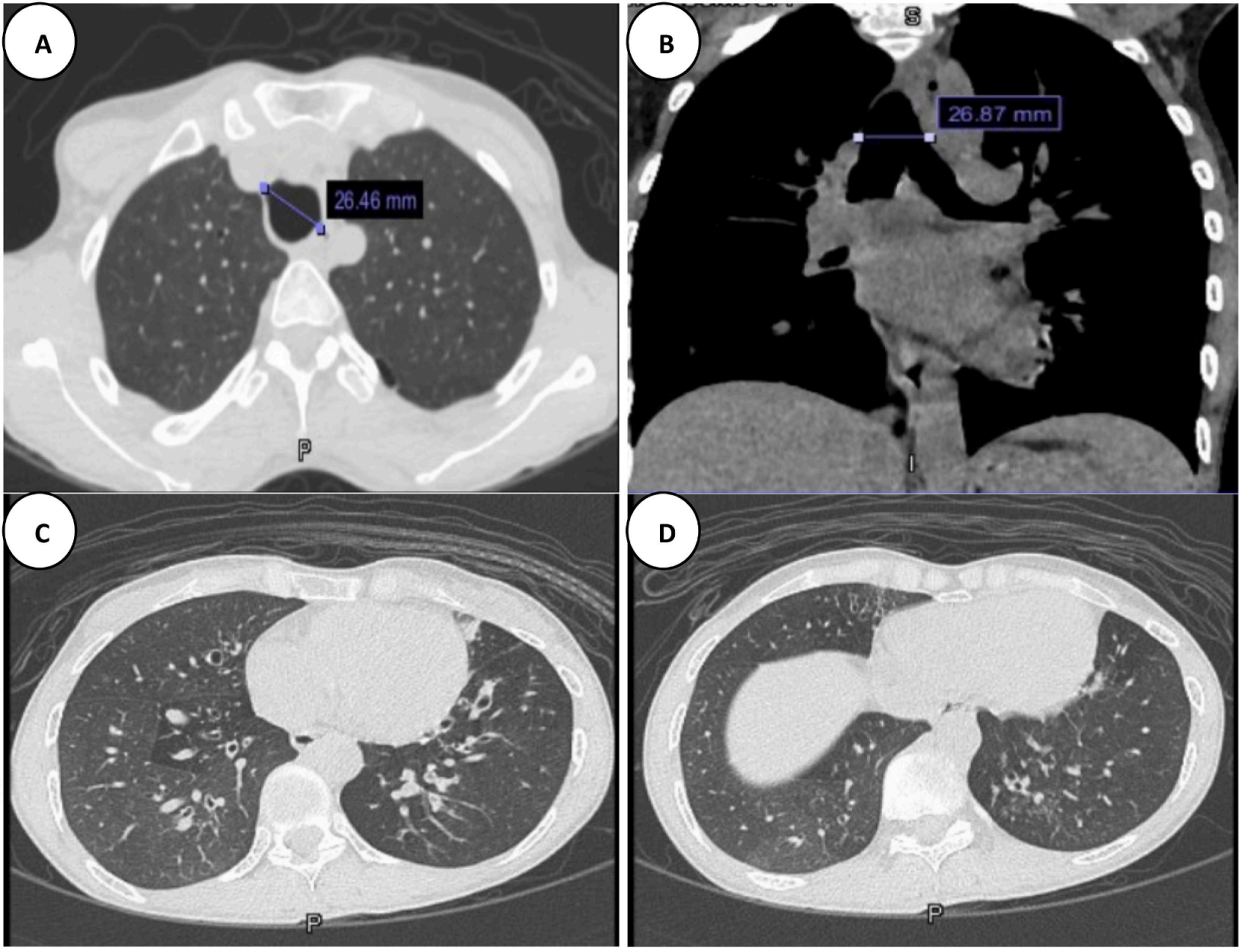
(A–D): Selected axial and coronal high-resolution CT images show marked dilatation of the trachea (arrow in A & B), dilatation of the main right and left bronchus (B), minimal paraseptal emphysema (A), and bilateral bronchiectasis (C & D).
